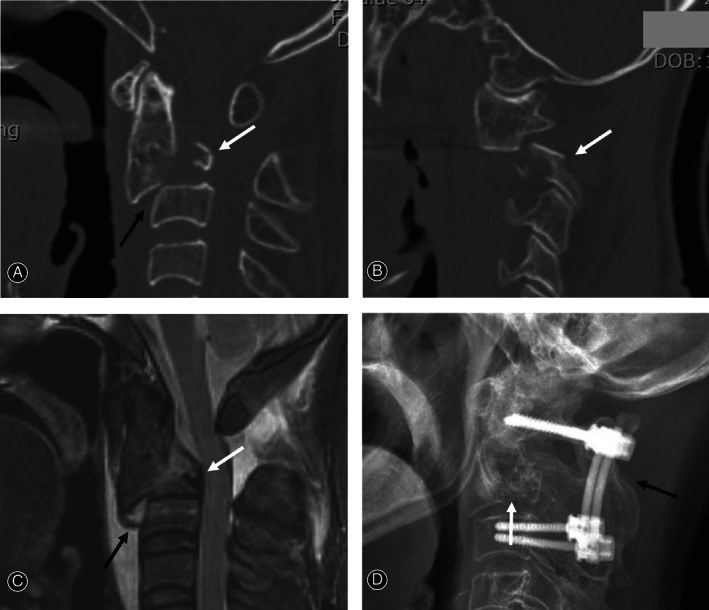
Sagittal and parasagittal computed tomography (CT) scans showing posterior tear drop (TD) fracture (white arrow) and C_2‐3_ anterior slip (dark arrow) (A) and hangman's fracture (white arrow) (B). Sagittal magnetic resonance imaging (MRI) (C) showing posterior TD fracture (white arrows) and complete C_2‐3_ discoligamentous injury including anterior longitudinal ligament, disc, and posterior longitudinal ligament at C_2‐3_ level (dark arrows). At 28 months follow‐up after surgery, lateral X‐ray (D) shows reduction of C_2‐3_ anterior slip and solid fusion of posterior C_1‐3_ fusion (dark arrow) and posterior TD fracture (white arrow).
